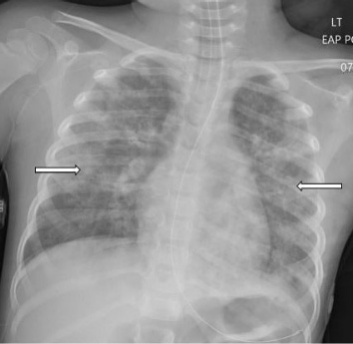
Chest radiograph on hospital day three showing worsening bilateral infiltrates and progression of mixed interstitial and alveolar opacities (arrows).
